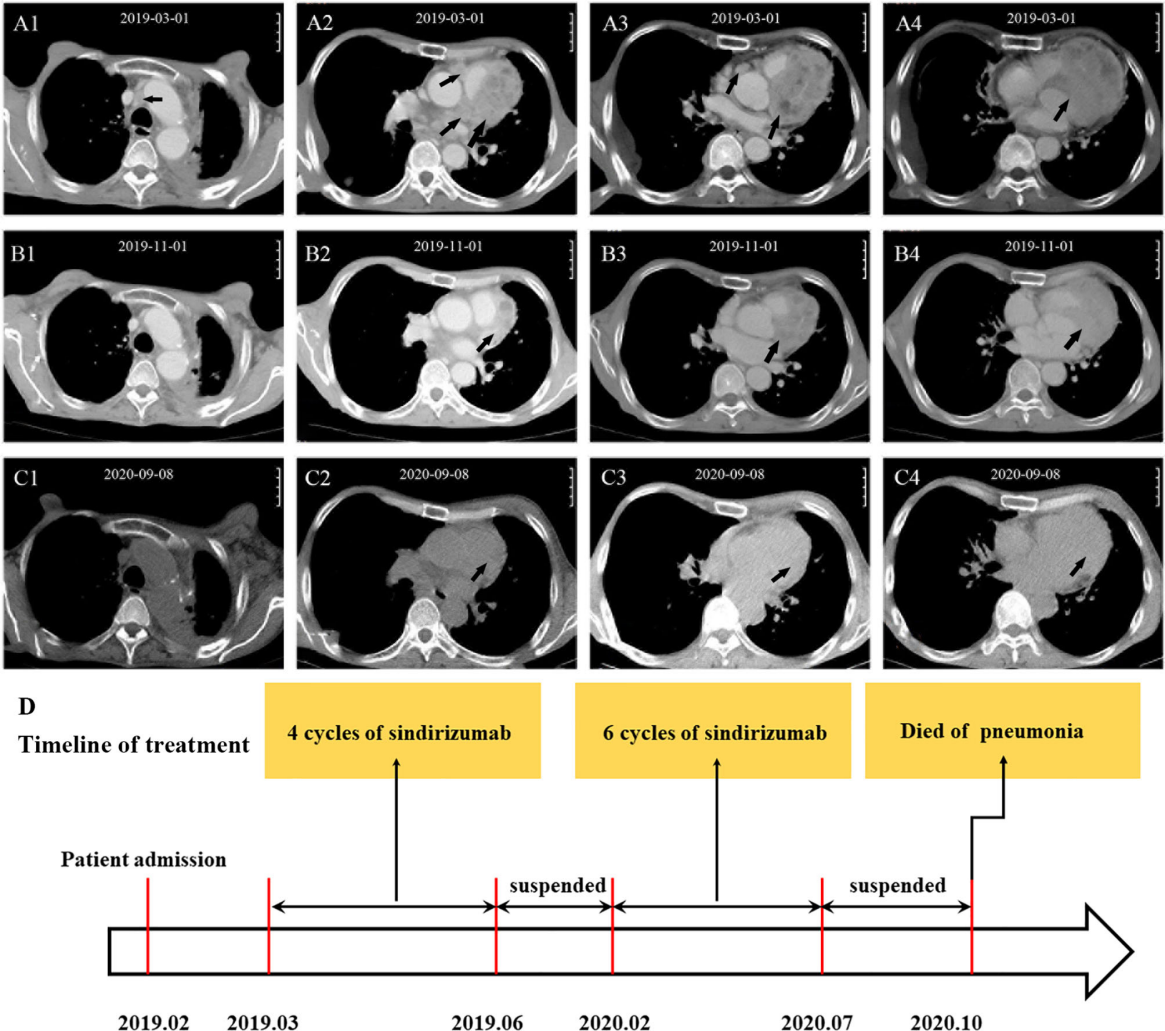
CT scans before and after treatment and timeline of treatment. Before treatment (A1–A4): The arrows show multiple lymph node shadows at the left hilum of the lung and mediastinum and a round, soft-tissue mass at the left edge of the Cardiac margin. After treatment (B1–B4): The arrows show that the enlarged mediastinal lymph nodes disappeared and myocardial metastasis significantly shrank. Follow-up (C1–C4): myocardial metastasis continuously shrank. Timeline of treatment of the patient (D): The arrow shows the whole process of diagnosis and treatment of the patient.
